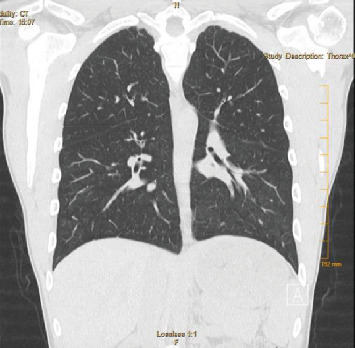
Case 2 chest CT scan 6 weeks post discharge (coronal view).
